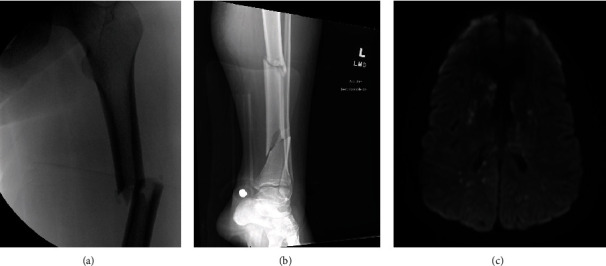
Patient imaging: (a) closed displaced left femur fracture; (b) closed displaced comminuted fracture of the left tibia; (c) MRI imaging demonstrating multiple hyperintense foci representing fat emboli.
